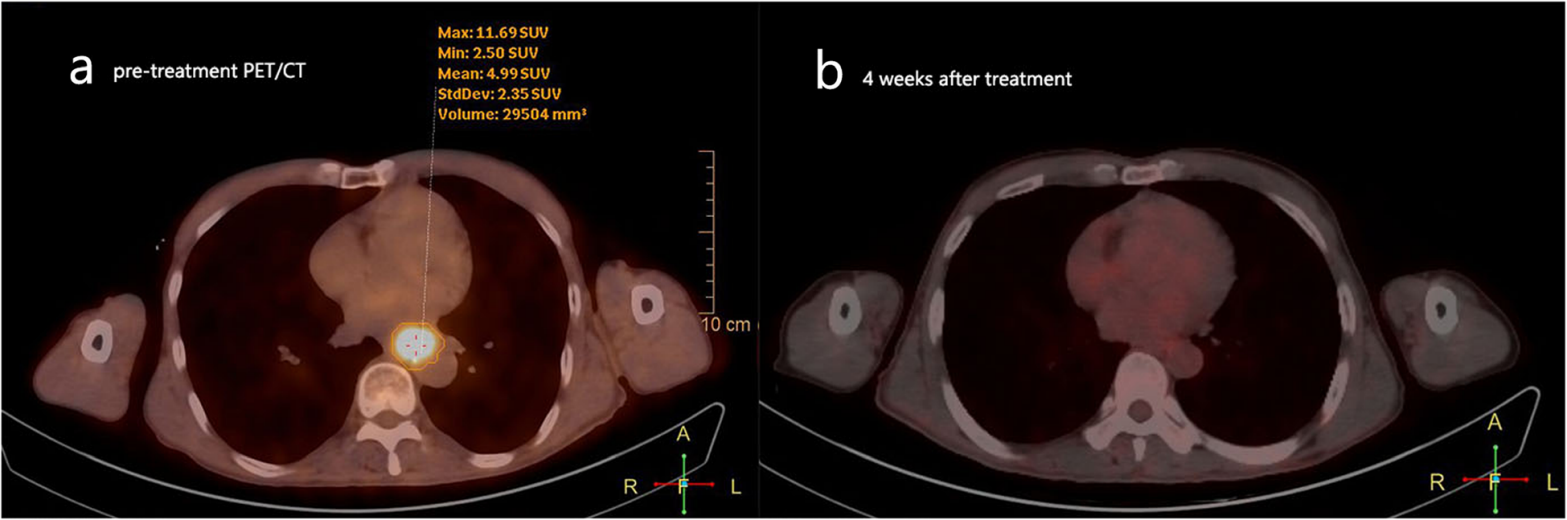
This is a stage III ESCC patient with a SUVmean value at 4.99 and the NLR value at 0.80. According to the prediction model, the patient has Y-value of 0.86, which belongs to a good response patient. After receiving CCRT and sequential 2 cycles of chemotherapy, the repeated PET/CT examination showed that the primary esophageal lesion completely disappeared, and the treatment response was CR according to RECIST (version 1.1) evaluation standard
